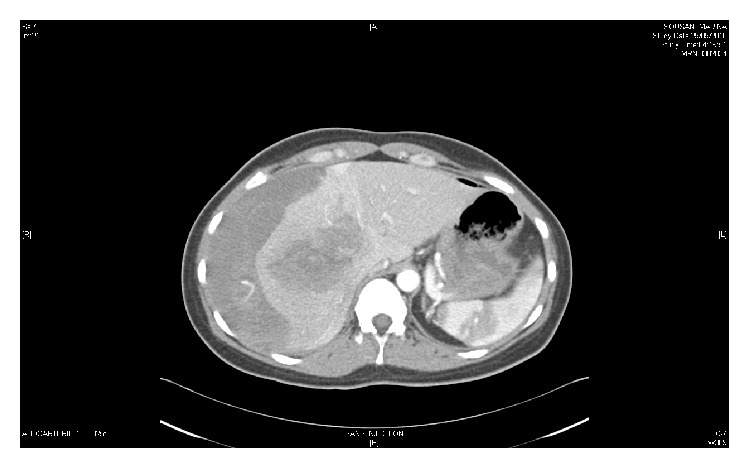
Subcapsular hematoma in abdominal CT scan without contraste.
